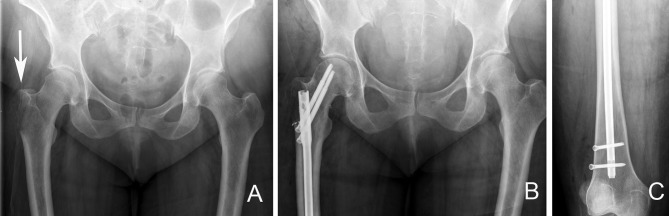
Right proximal femur bone metastasis treated with a locked intramedullary nail. (A). Destructive lytic lesion involving the proximal femur greater trochanter area (arrow). (B) Postoperative X-ray after fixation with a cephalomedullary nail. Note the proximal fixation spanning the femoral head and neck. (C) Distal locking bolt fixation to ensure axial and rotational stability.
